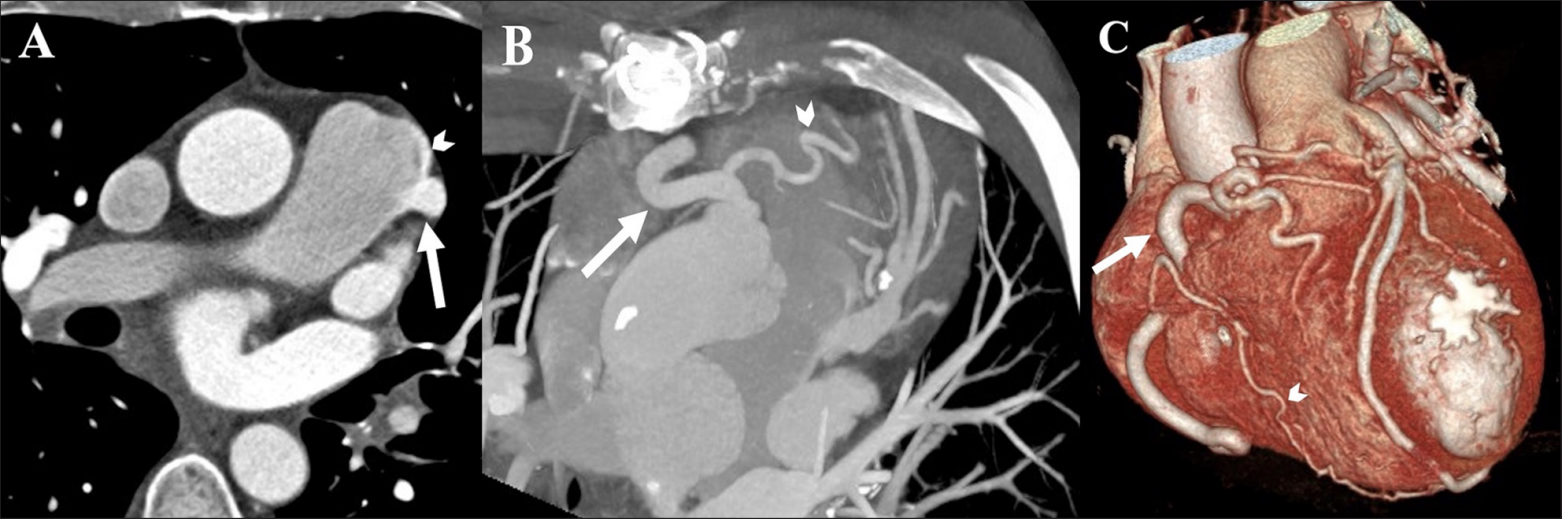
The CT demonstrated multiple areas of myocardial and subendocardial infarcts and a large left ventricle apex. A mitral mechanic valve was also depicted (Figure 2). These findings were consistent with an adult type of anomalous origin of the LCA from the pulmonary artery (ALCAPA) syndrome. The patient was treated only for her PAF by invasive intervention, and no surgical procedure was performed.
